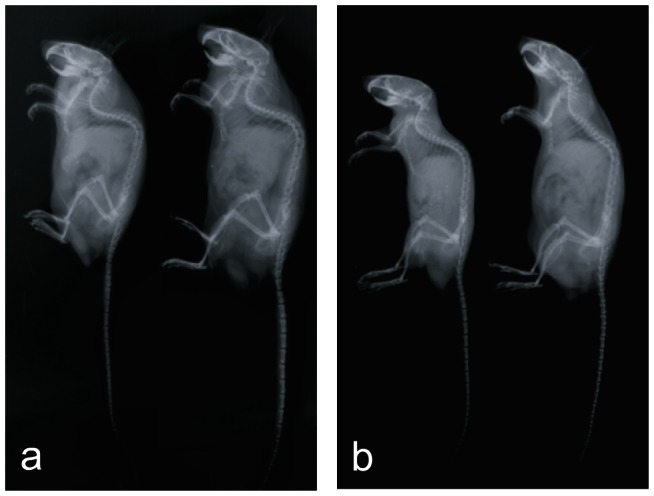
Radiographic analysis of 6 month old male TrkB mutant and control littermate (A), and p38α mutant and control littermate (B). Exaggerated kyphosis is post-mortem.
